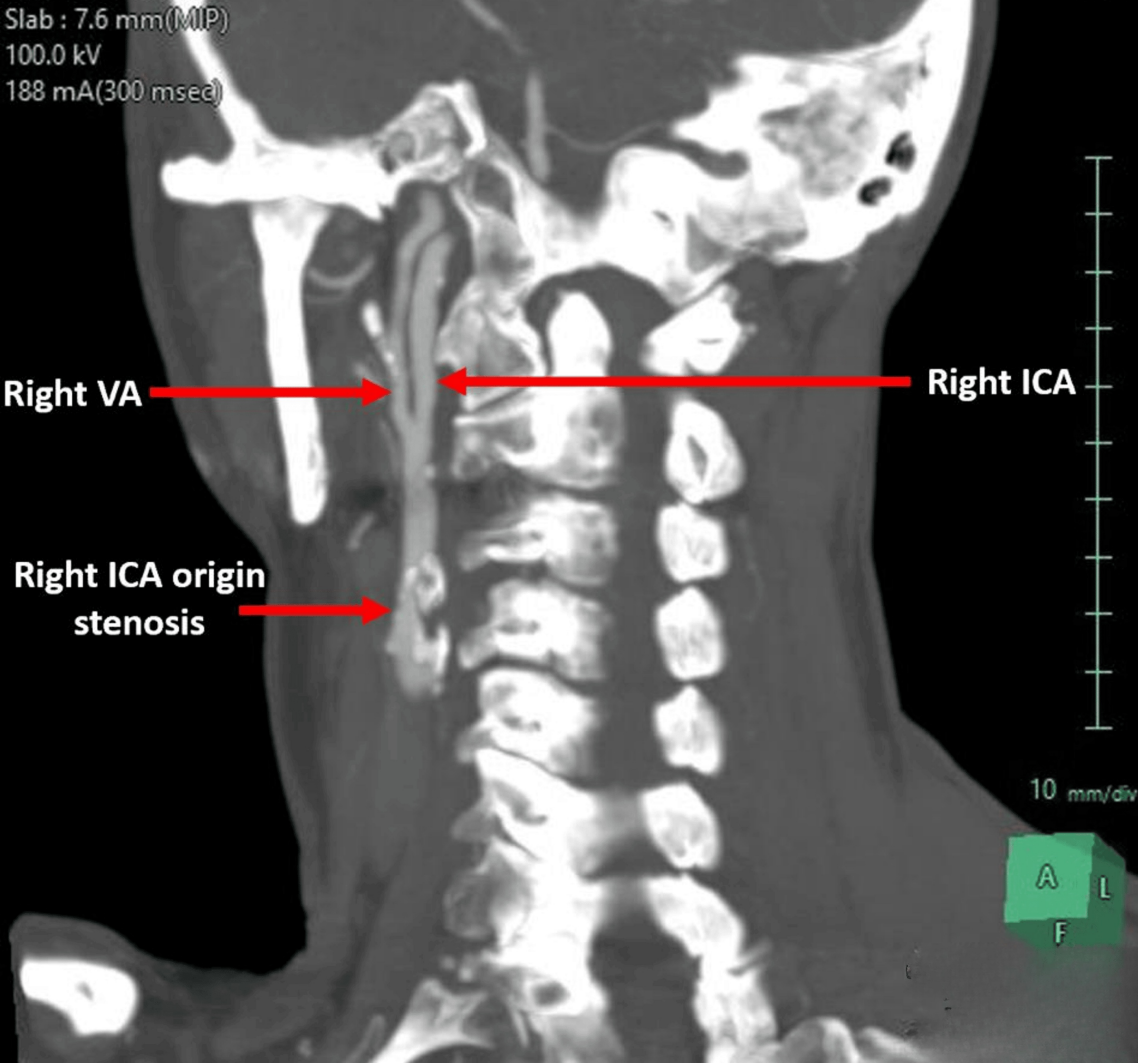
CTA demonstrating the main right VA arising from the right distal ICA with stenosis in the ICA CTA: computed tomographic angiography, VA: vertebral artery, ICA: internal carotid artery
